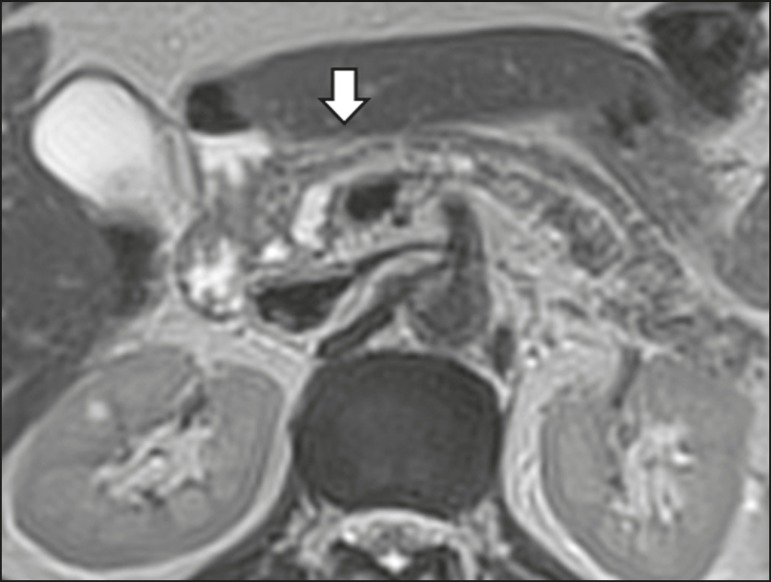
T2-weighted MRI sequence showing dilation of the pancreatic duct (arrows)
